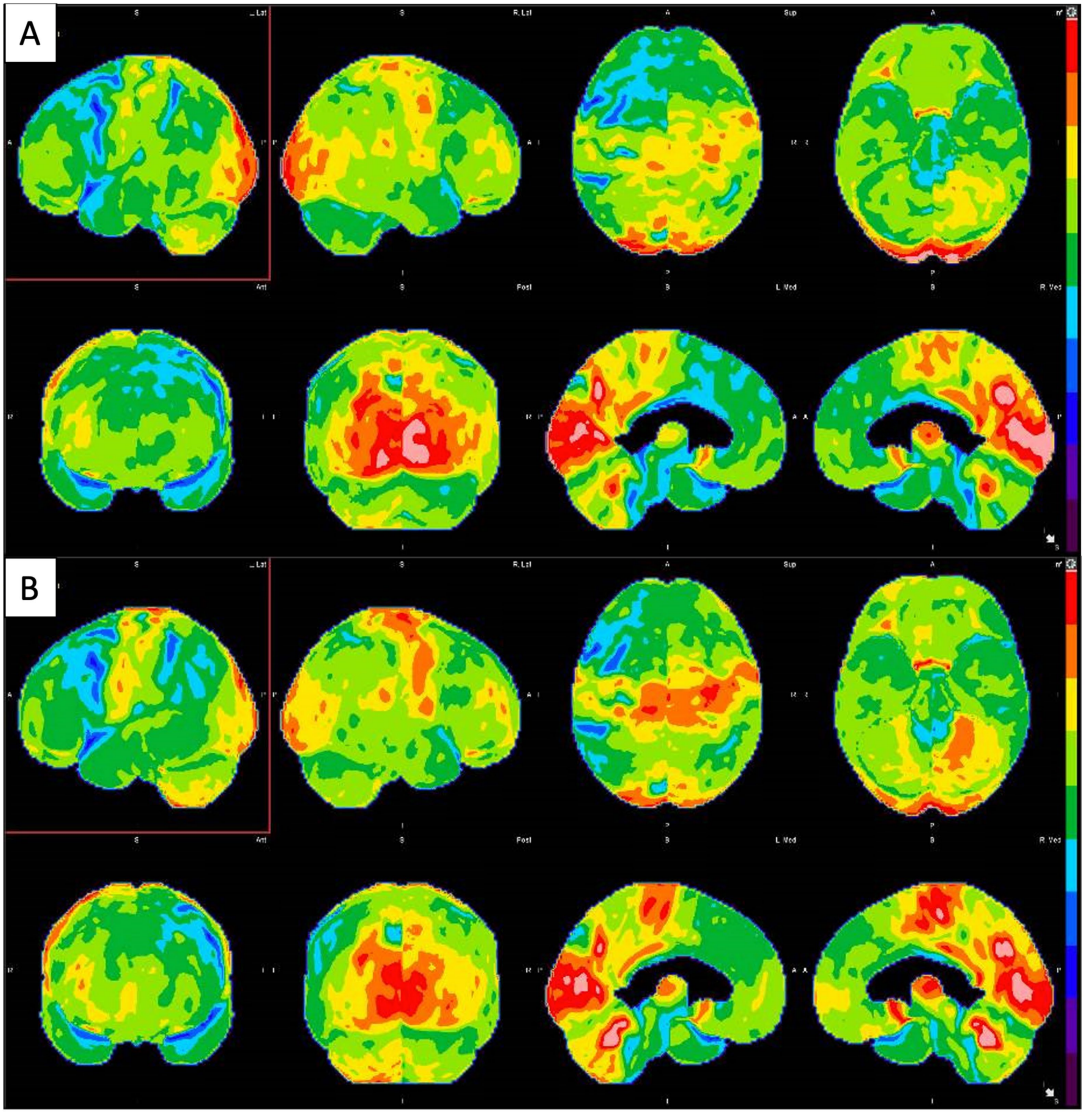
(A) Brain FDG-PET imaging: Three-dimensional stereotactic surface projection (SSP) maps demonstrate severe asymmetric hypometabolism in the left frontal lobe, particularly involving the left dorsolateral and fronto-insular region, including the left frontal operculum (pars opercularis and pars triangularis of the left inferior frontal gyrus, expected Broca’s area). There is accompanying crossed cerebellar diaschisis, with right greater than left cerebellar hypometabolism. (B) Second Brain FDG-PET imaging taken 12 months after the first showed similar hypometabolism distributions.
